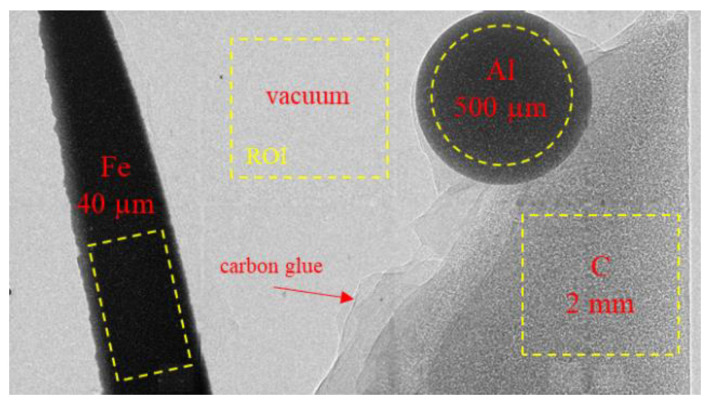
Radiographic image of a test object made of pure iron, aluminum and carbon to determine CNR. The marked areas in yellow are used for evaluation and the values correspond to element thickness. The aluminum ball is fixed to the carbon surface using carbon glue as indicated with the red arrow.
